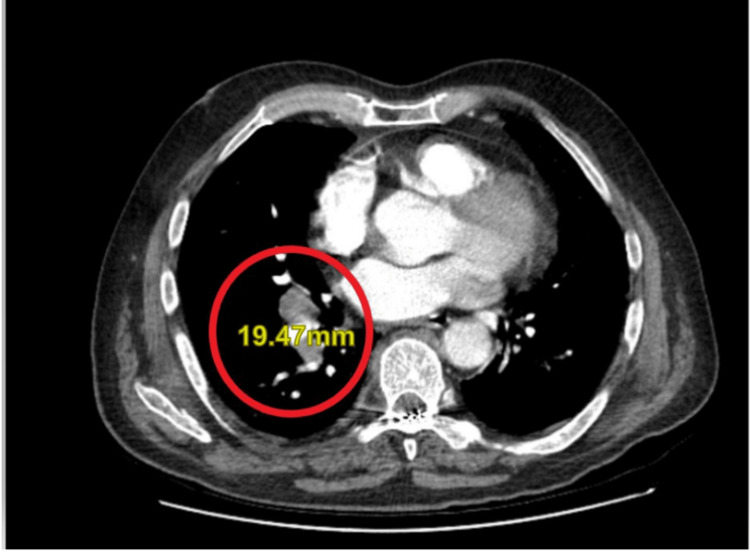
CT angiogram revealing right hilar lymph node measuring 1.9 cm.
